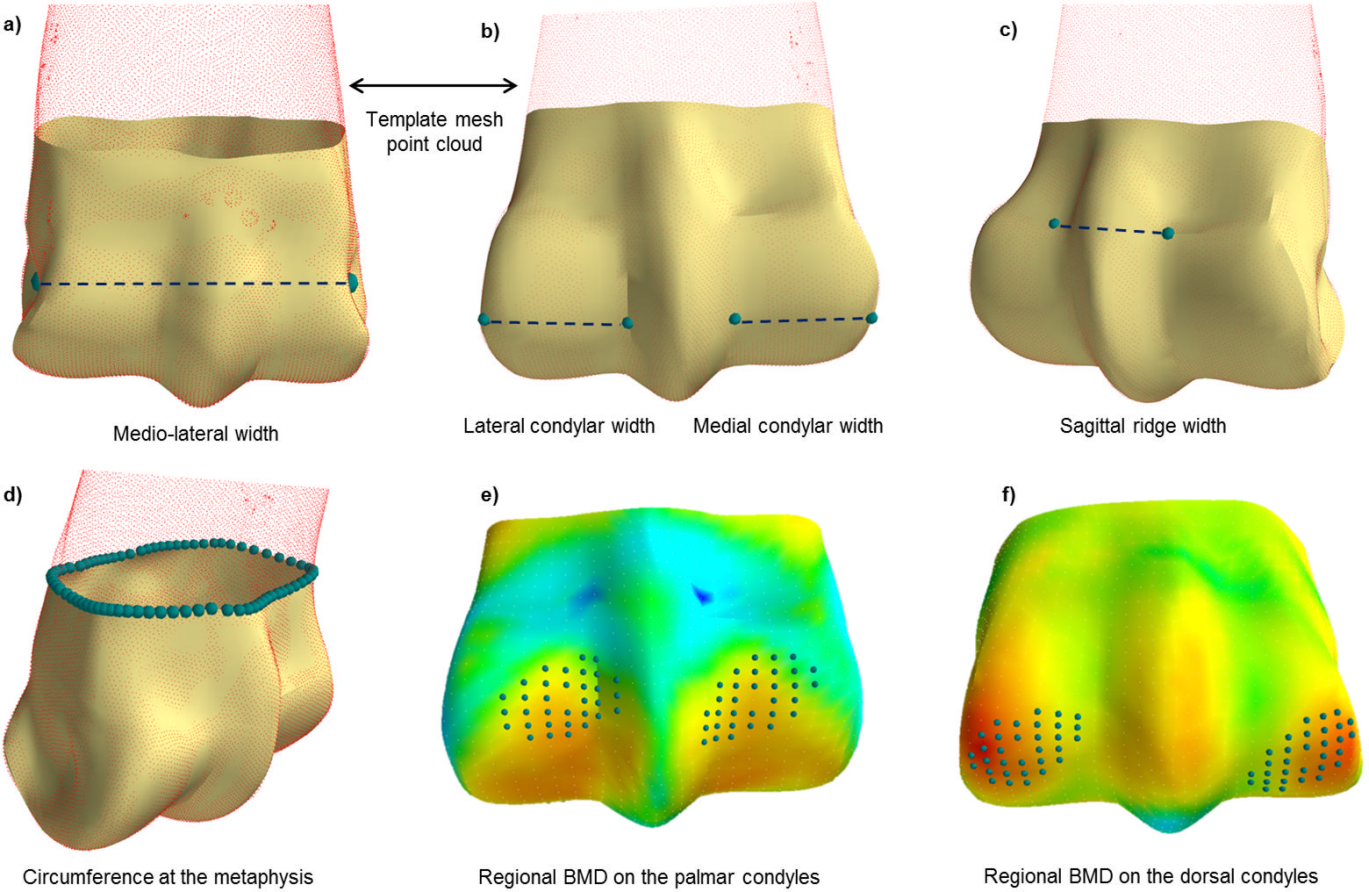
Initial estimates of third metacarpal bone shape and parameters to quantify changes observed in the principal component analysis, the segmented point cloud from the original CT data is included as a reference. Large blue points indicate anatomical landmarks identified from a template mesh and subsequently fit to the population to measure morphological parameters.
